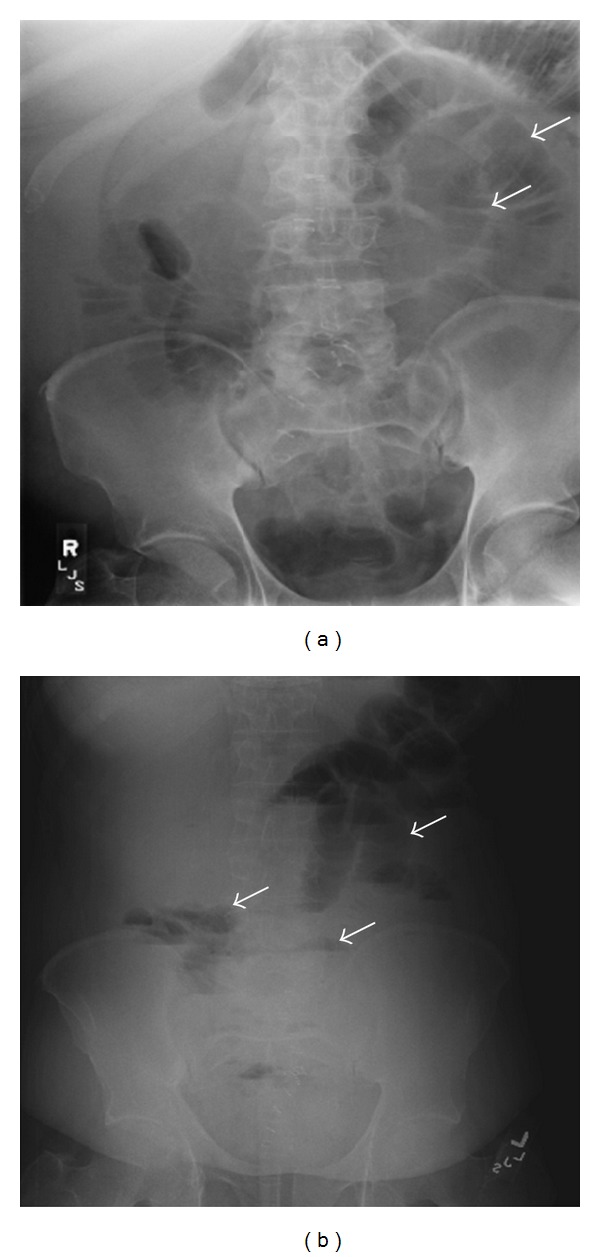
X-ray abdomen. (a) Supine abdominal radiograph shows dilated small bowel loops and absence or paucity of gas in the large bowel due to distal small bowel obstruction. (b) Upright abdominal radiograph shows multiple air-fluid levels in upper and central abdomen that represent dilated proximal and central fluid-filled small bowel due to distal small bowel obstruction (arrows).
